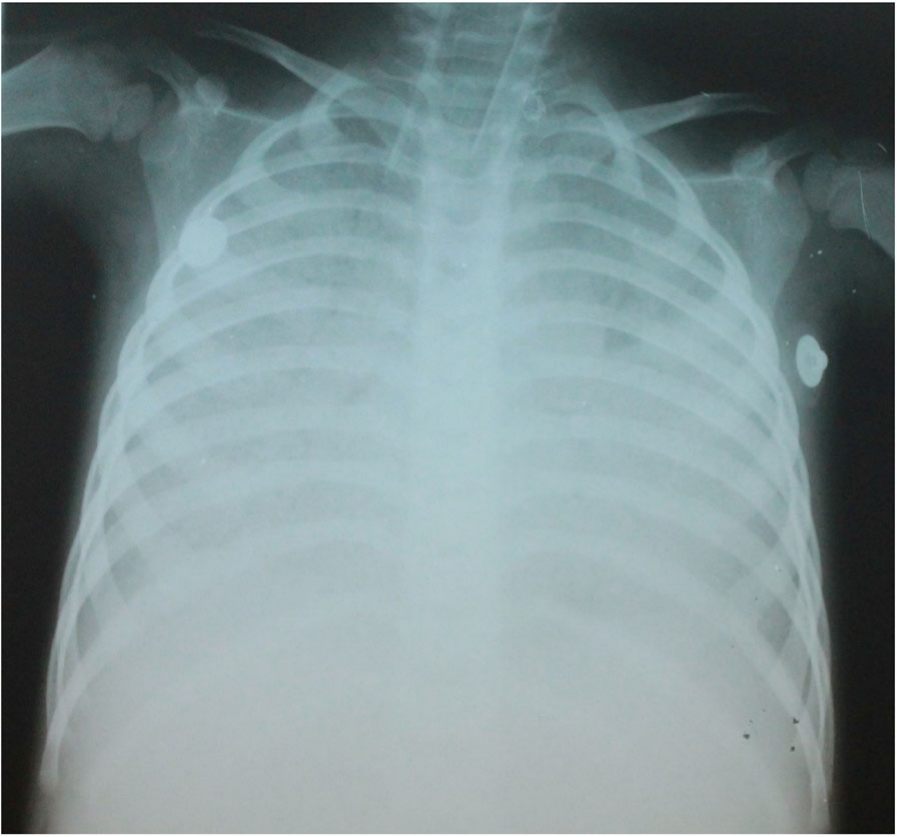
Chest X-ray on admission to PICU
